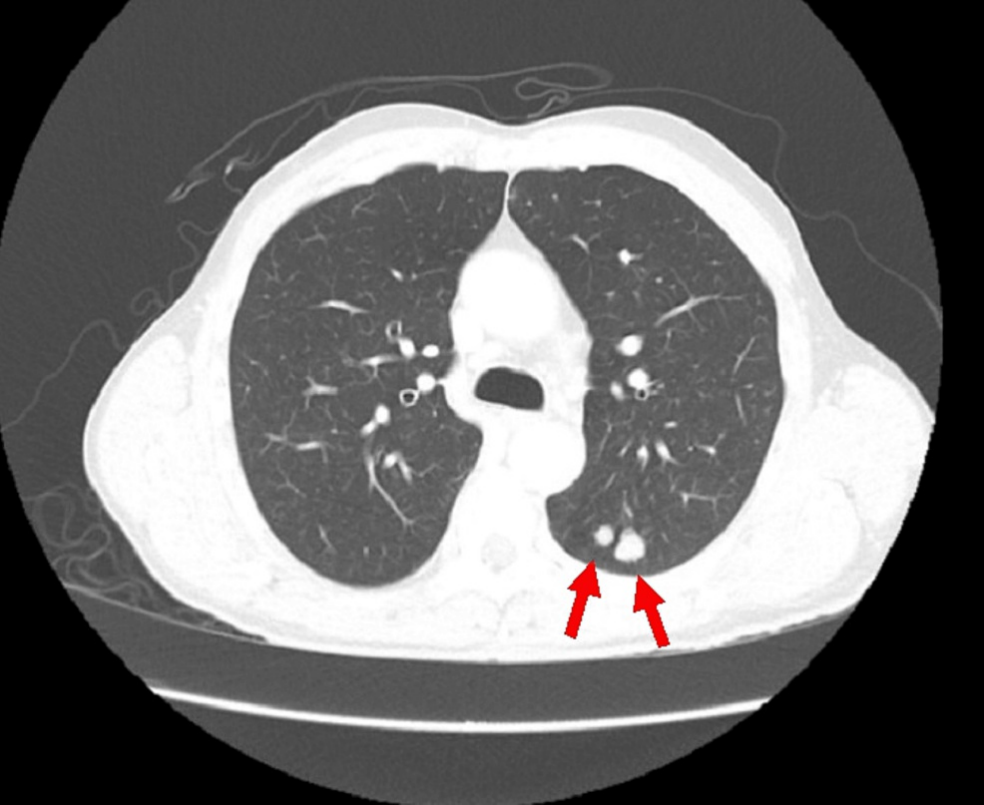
Initial CT chest with IV contrast. CT chest showing two left posterior pulmonary nodules measuring 7 and 12 mm in size (red arrows). CT: computed tomography; IV: intravenous.
